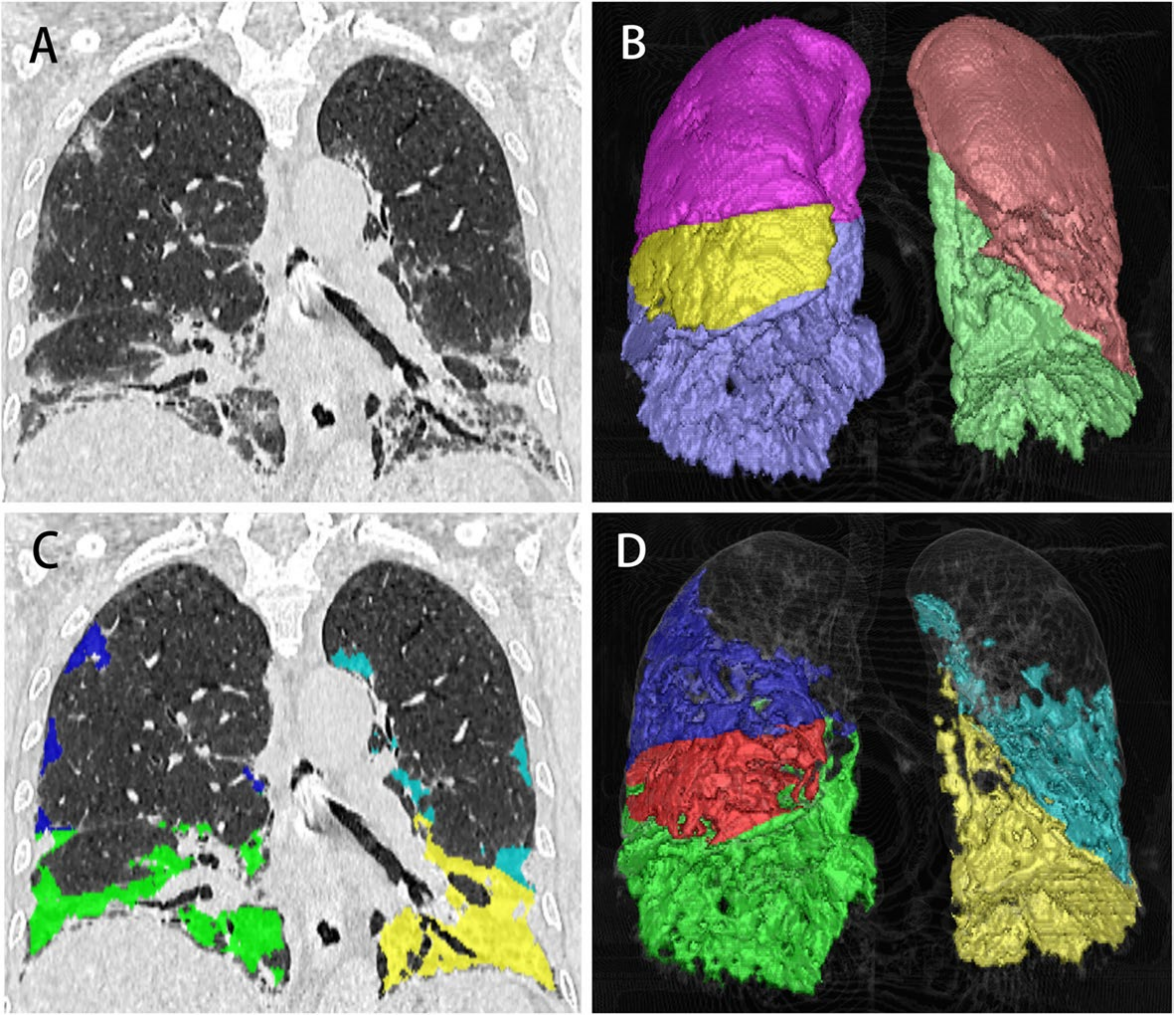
The segmentation of infected areas (i.e., the region of interest, ROI) on the FACT Medical Imaging System. A 59-year-old female with anti-synthetase syndrome and a long history of corticosteroid use was admitted to hospital with fever and cough for 7 days. A Sagittal HRCT images showed ground-glass opacity and thickening interlobular septal predominantly in both lower lungs. B Three-dimensional (3D) volume rendering image of the lobes (purple: right upper lobe; yellow: right middle lobe; blue: right lower lobe, brown: left upper lobe; green: left lower lobe). C and D Sagittal CT and 3D images of automatically identified infected areas. Different colors indicated that the infection was in different lobes (blue: right upper lobe; red: right middle lobe; green: right lower lobe; cyan: left upper lobe; yellow: left lower lobe). The ROIs are integrated as one and radiomic features were extracted from it
